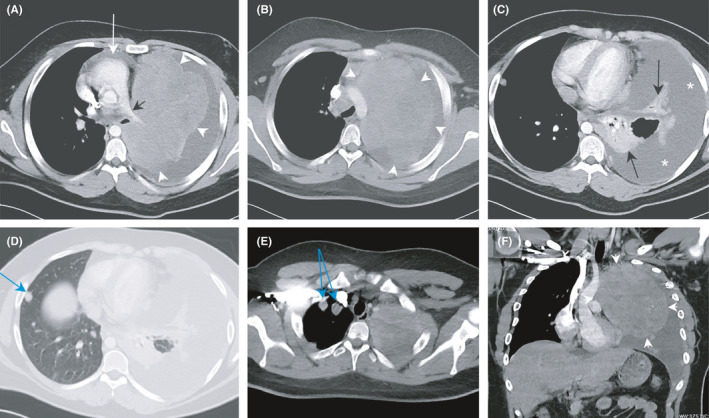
(A and B) Axial images of the contrast‐enhanced CT chest. A mass lesion can be seen in the left hemithorax (between white arrowheads), which is attenuating the left main pulmonary artery (black arrow) and causing a shift of the mediastinum to the right (white arrow). (C) An almost total collapse of the left lung (black arrows) and sizable left pleural effusion (white asterisk). (D and E) Multiple pleural‐based nodular lesions noted in the right hemithorax (blue arrows). (F) Coronal reformat image of the contrast‐enhanced CT chest
